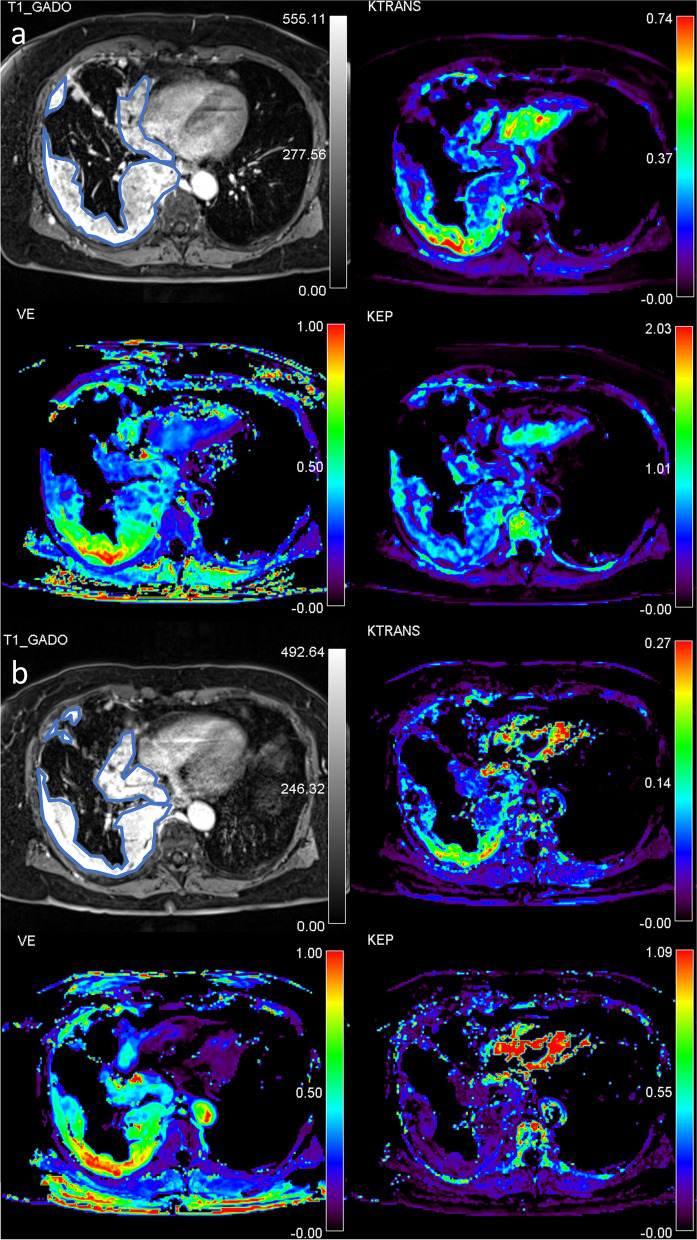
An example of a patient with long PFS and OS. Pre-treatment and intra-treatment DCE-MRI (a and b), a post-contrast T1 weighted-image is shown together with ET-calculated *K*^trans^, v_e_ and k_ep_ parametric maps. Regions of interest (ROI) are drawn around the MPM periphery on post-contrast T1 weighted-image. The pre-treatment median values were: *K*^trans^ = 0.22 min^-1^, v_e_ = 40 ml/100ml, and k_ep_ = 0.54 min^-1^ and the intra-treatment values were *K*^trans^ = 0.18 min^-1^, v_e_ = 48 ml/100ml, and k_ep_ = 0.39 min^-1^. The parametric maps show MPM spatial heterogeneity regarding its vascular properties
